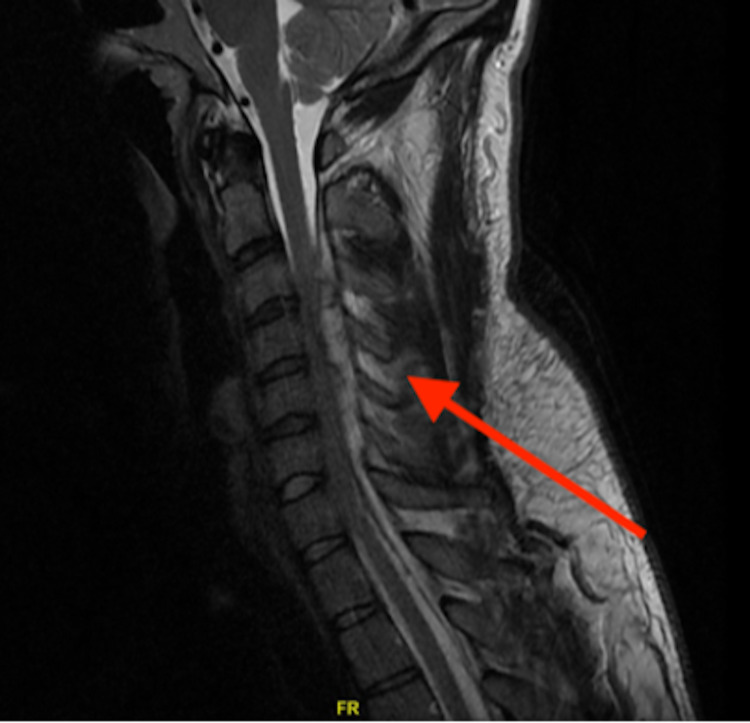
Sagittal T2 enhanced cervical MRI showing posterior epidural heterogenous collection representing an epidural hematoma extending from the C2-3 level to the T3 level with moderate flattening of the cord
